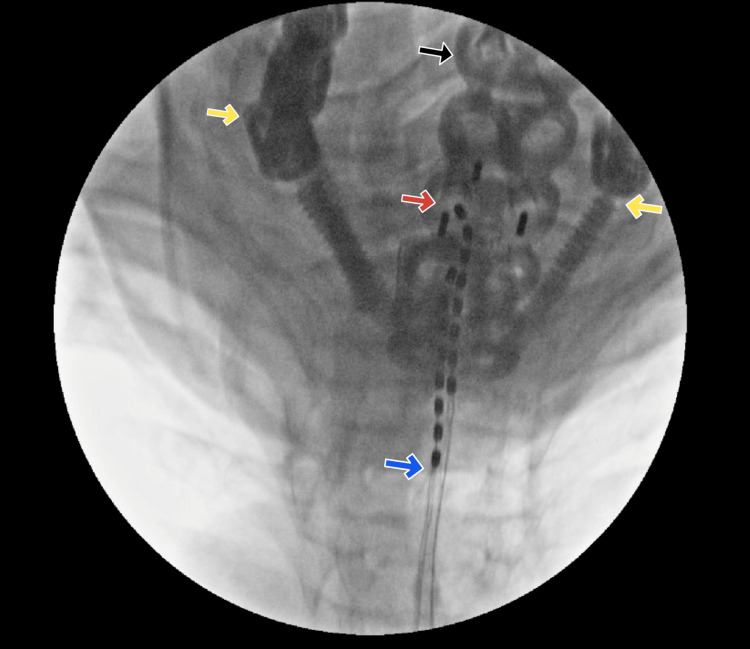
Fluoroscopic radiograph status-post SCS implantation Posterior-anterior fluoroscopic radiograph taken at the completion of SCS implantation. The blue arrow marks the inferior end of the SCS electrode; the red arrow marks the superior end (left at T1, right at C7 superiorly). The inferior ends of posterior fusion screws are indicated with yellow arrows bilaterally. The black arrow points to the plate from anterior cervical discectomy and fusion. This image highlights the successful implantation of SCS leads in a region of the cervical spine with significant overlying surgical hardware.
